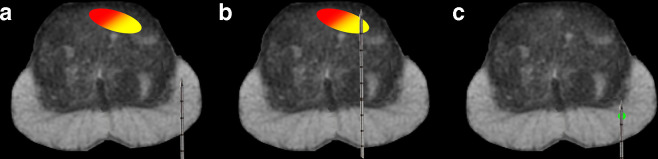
Limitations of TRUS-guided biopsy. Clinically significant tumors may not be detected (a), the tumor grade may be underestimated (b), and clinically insignificant tumors may be overdiagnosed (c). TRUS,transrectal ultrasound.
